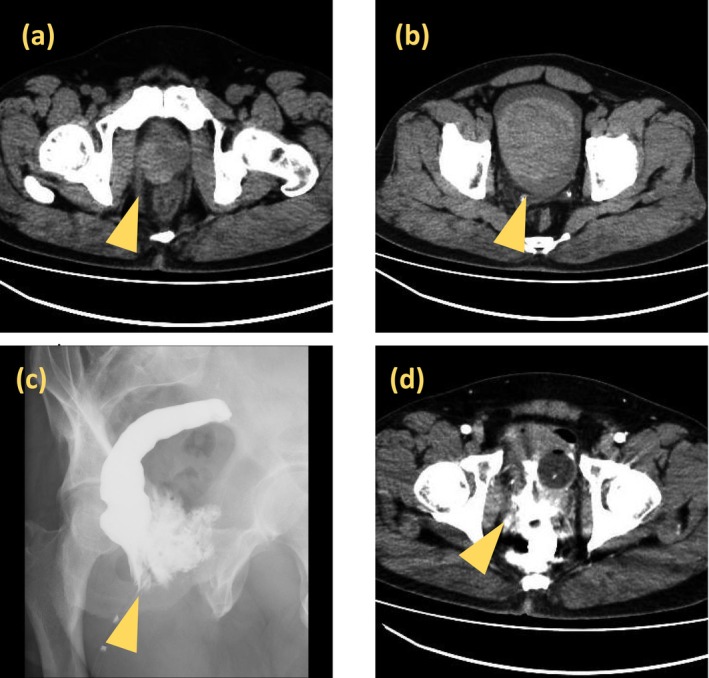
(a) Non‐contrast CT showing a high‐density area (approximately 4 cm) between the right anterior wall of the rectum and the right dorsolateral prostate, suggestive of hematoma. (b) CT revealing a high‐density area (approximately 10 cm) within the bladder, also indicative of hematoma. (c) CE demonstrating extravasation of contrast medium outside the rectal lumen without bladder visualization. (d) Post‐enema CT showing high‐density contrast accumulation around the rectum, prostate, bladder, and perivesical space, raising suspicion of combined rectal and bladder injury without evidence of intraperitoneal leakage.
